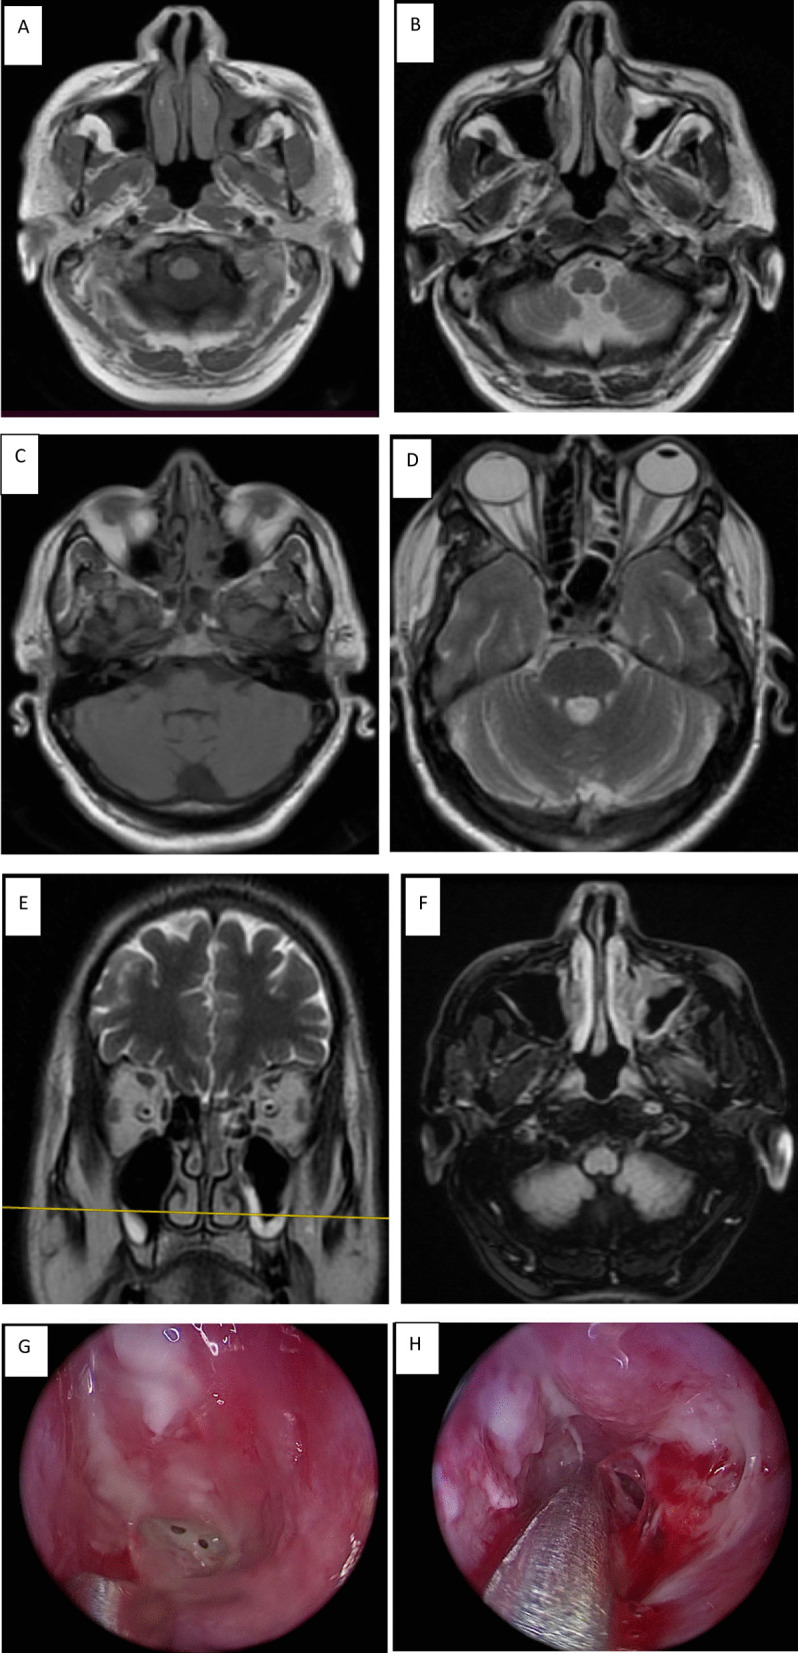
A 62-year-old diabetic female patient presented with facial tingling after 2 weeks of COVID-19 infection. A, C MRI axial T1WI B, D MRI axial T2WI, E coronal T2WI and F axial fat suppression images show subtle mucosal thickening of left maxillary sinus, left nasal cavity, and ethmoidal air cells without extension beyond the sinonasal area. Clinical examination revealed pale mucosa. Endoscopic debridement was done based on clinical suspicious and strong positive history. Fungal culture revealed definitive Mucormycosis infection. G, H 0-degree endoscopic views show pale oedematous mucosa with early necrosis of the left middle meatus
